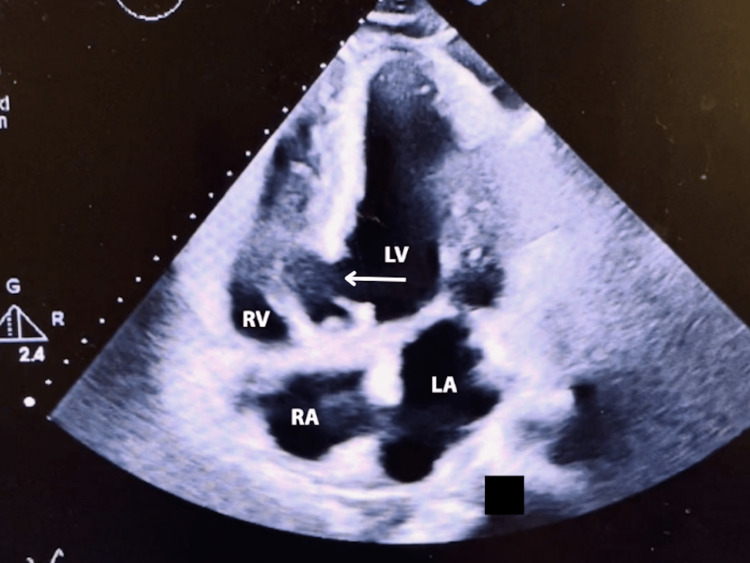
Transthoracic Echocardiogram demonstrating muscular wall defect in the left ventricular septum (arrow)
